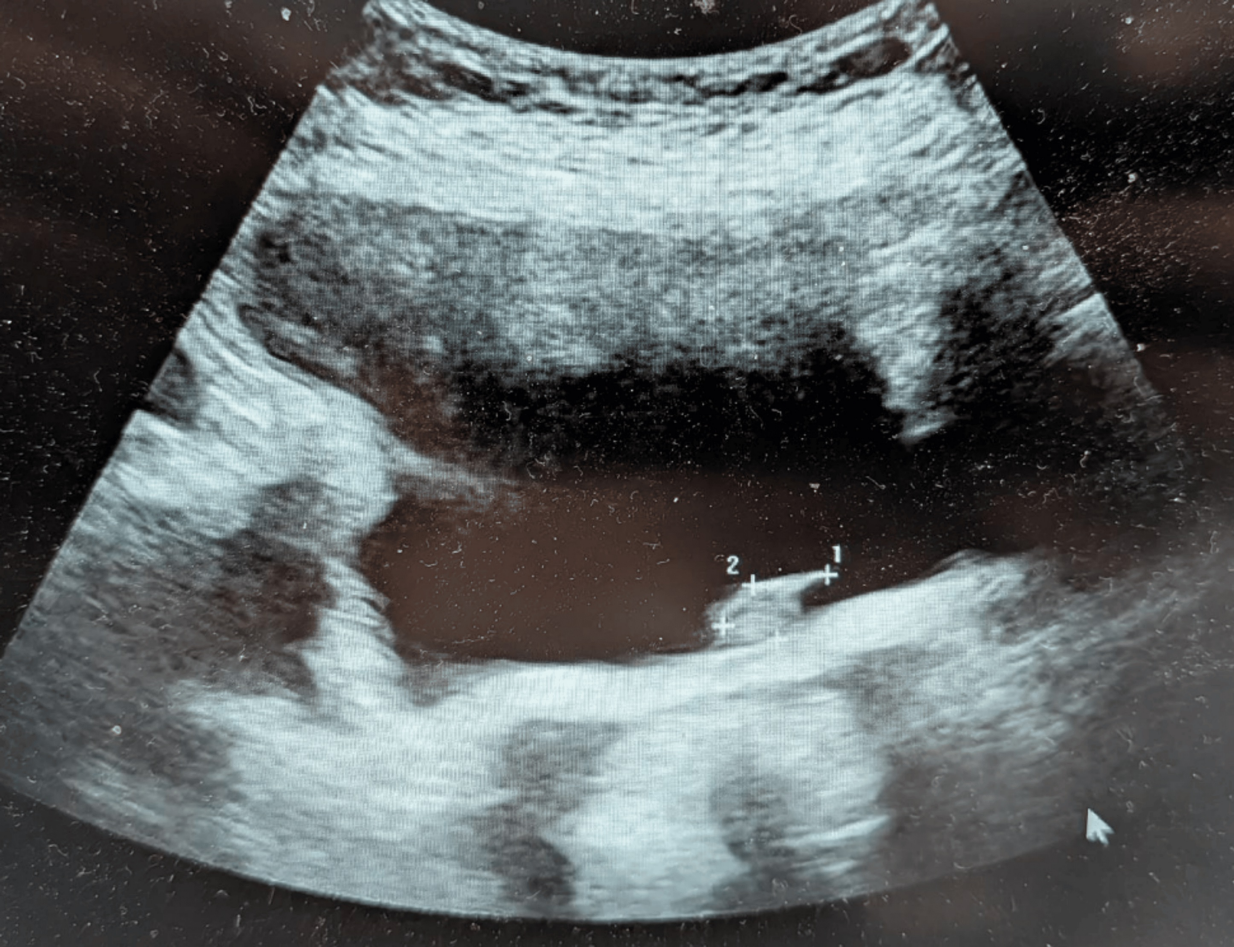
Ultrasound bladder showing bladder lesion (Case 4)
